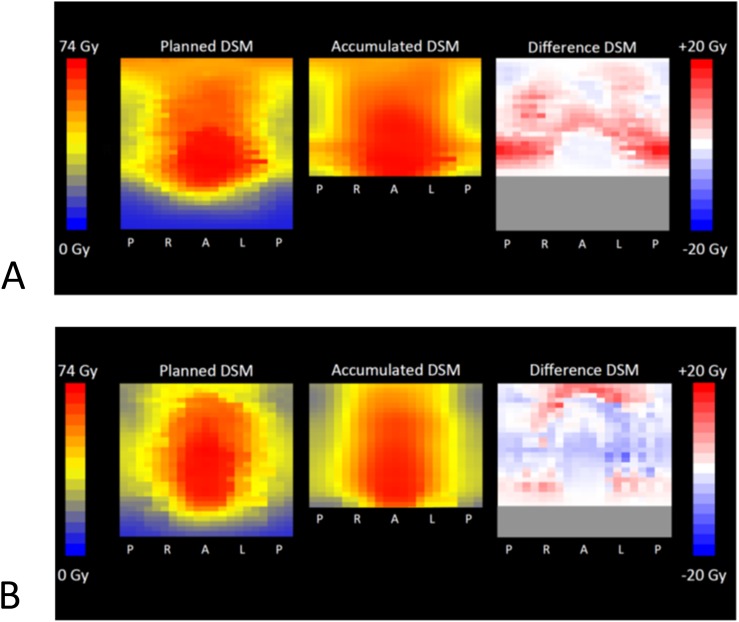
Dose–surface maps (DSMs) for Patient A, with the highest accumulated equivalent uniform dose (EUD) compared with planned (+5.3 Gy) of the 10 patients and for Patient B with the lowest accumulated EUD compared with planned (−10.2 Gy). The rectum was considered a cylinder, and daily delivered dose was sampled at a set of equally spaced points on each MV slice. The cylinder was then “cut” at the point where a vertical line from the centroid of each outline crossed the posterior edge and unfolded. The DSMs were summed over all the fractions, based on the superior–inferior positions of each image corrected for the shifts applied at treatment. Results are shown as accumulated DSMs; planned DSMs are shown for comparison. The difference DSM represents the difference for each pixel between accumulated and planned dose. Since the length of the MV CT image set was less than that of the rectum, the difference DSM is shorter (shown in grey). Although Patient A had a median *D*_A_ of 1.7 Gy higher than planned, areas of the superior rectum received doses of up to 2.8 Gy less than planned. Patient B had a median *D*_A_ of −0.8 Gy compared with that planned; in this case, inferior and superior rectum received up to 13.9 Gy more than planned. A, anterior; L, left; P, posterior; R, right. Reproduced from Scaife et al^122^ with permission from the British Institute of Radiology.
